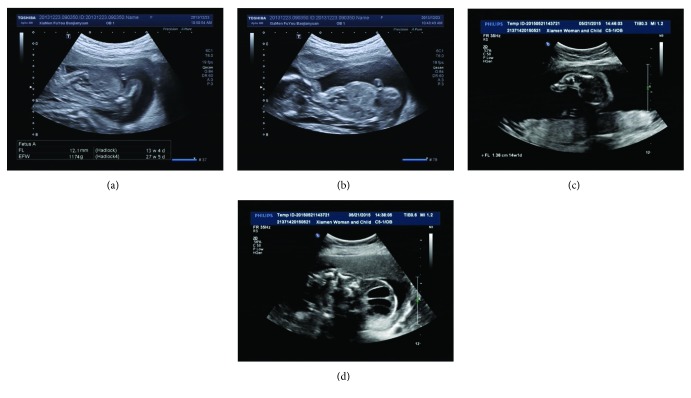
(a) Case 1: sonogram of femur length, which is extremely short. (b) Case 1: ultrasound scans showing narrow thorax and protuberant abdomen. (c) Case 2: sonogram of femur length, which is extremely short. (d) Case 2: ultrasound scans showing a large cystic hygroma.
